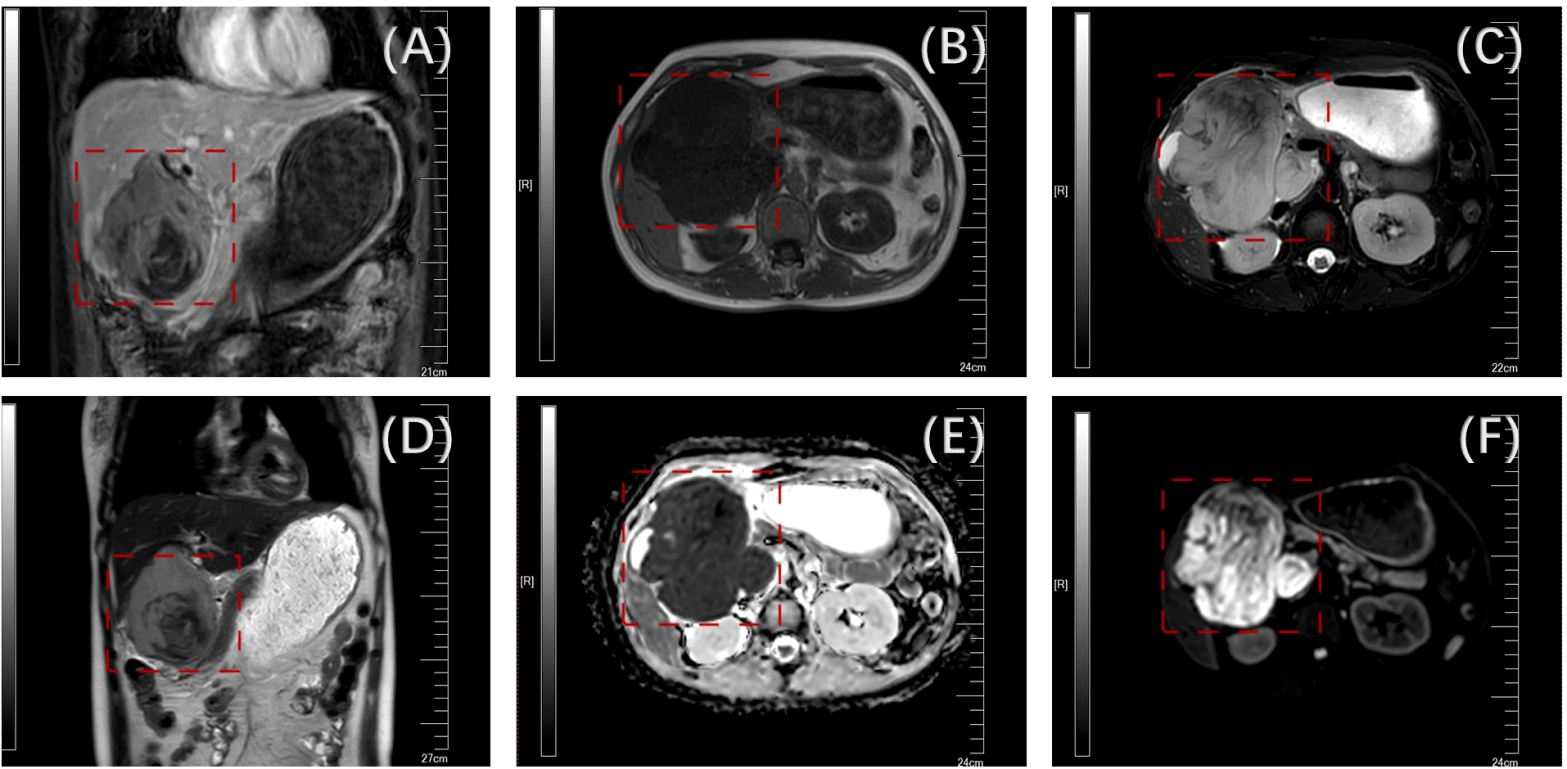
Preoperative enhanced nuclear magnetic examination(the tumor is boxed) [(A) mDIXON; (B) sF; (C) DWI; (D) T2W; (E) dADC; (F) T2].
